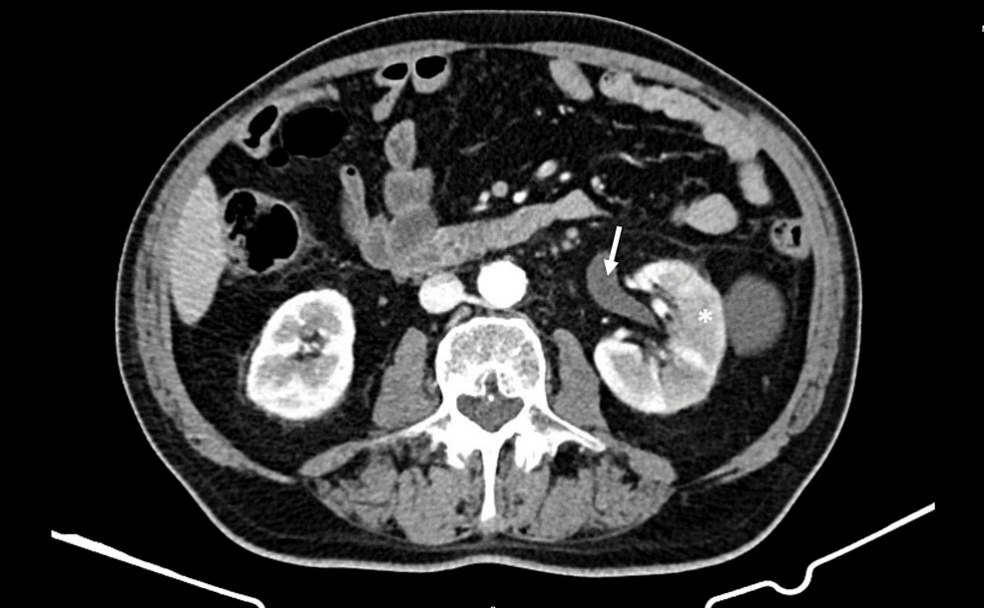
Abdominal CT scan showing areas of contrast attenuation in renal parenchyma (asterisk) and hydronephrosis of the left kidney (arrow)
